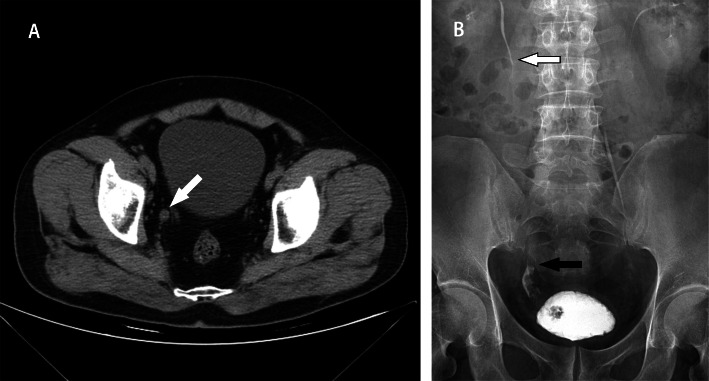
Computed tomography and intravenous urogram findings (a) Computed tomography shows an enlargement in the inferior part of the right ureter with a suspected solid mass; (b) Intravenous urogram shows a “sponge-like” filling defect in the right distal dilated ureteral lumen (black arrow) without dilation of the upper urinary tract and renal pelvis (white arrow), and an oval-shaped filling defect of the ureteral orifice inside the bladder
